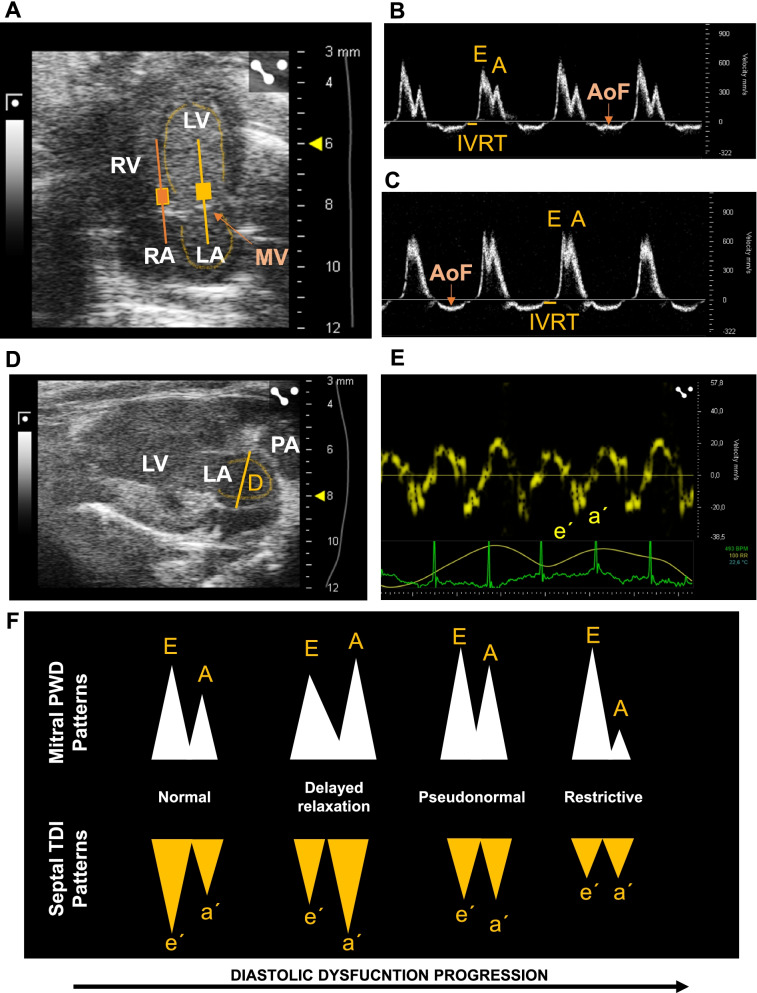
Echocardiography measures used to assess diastolic dysfunction and its progression. A Bidimensional apical 4-chamber view highlighting the visualized structures and the sample volume position to obtain a mitral inflow pulse wave Doppler echocardiography (PWD, yellow filled square) and a tissue Doppler image (orange filled square). B, C Normal (B) and abnormal (C) mitral inflow PWD showing the early (E) and late (A) wave peak velocity and the isovolumetric relaxation time (IVRT). D Bidimensional LAX view optimized to visualize the left atrial (LA) to obtain a mediolateral end-systolic diameter (D). E Tissue Doppler imaging at the septal corner of the mitral annulus to obtain early and passive filling myocardial velocities (e′ and a′, respectively). F Representative mitral PWD and tissue Doppler waves change as diastolic dysfunction progresses. LV, left ventricle; LA, left atrium, RV, right ventricle; RA; right atrium; MV, mitral valve; E, early wave peak velocity; (A) late wave peak velocity; IVRT, isovolumetric relaxation time; AoF, aortic flow; PA, pulmonary artery; D, diameter; E′, early filling velocity; A′, passive filling velocity; PWD, pulse wave Doppler; TDI, Tissue Doppler imaging
